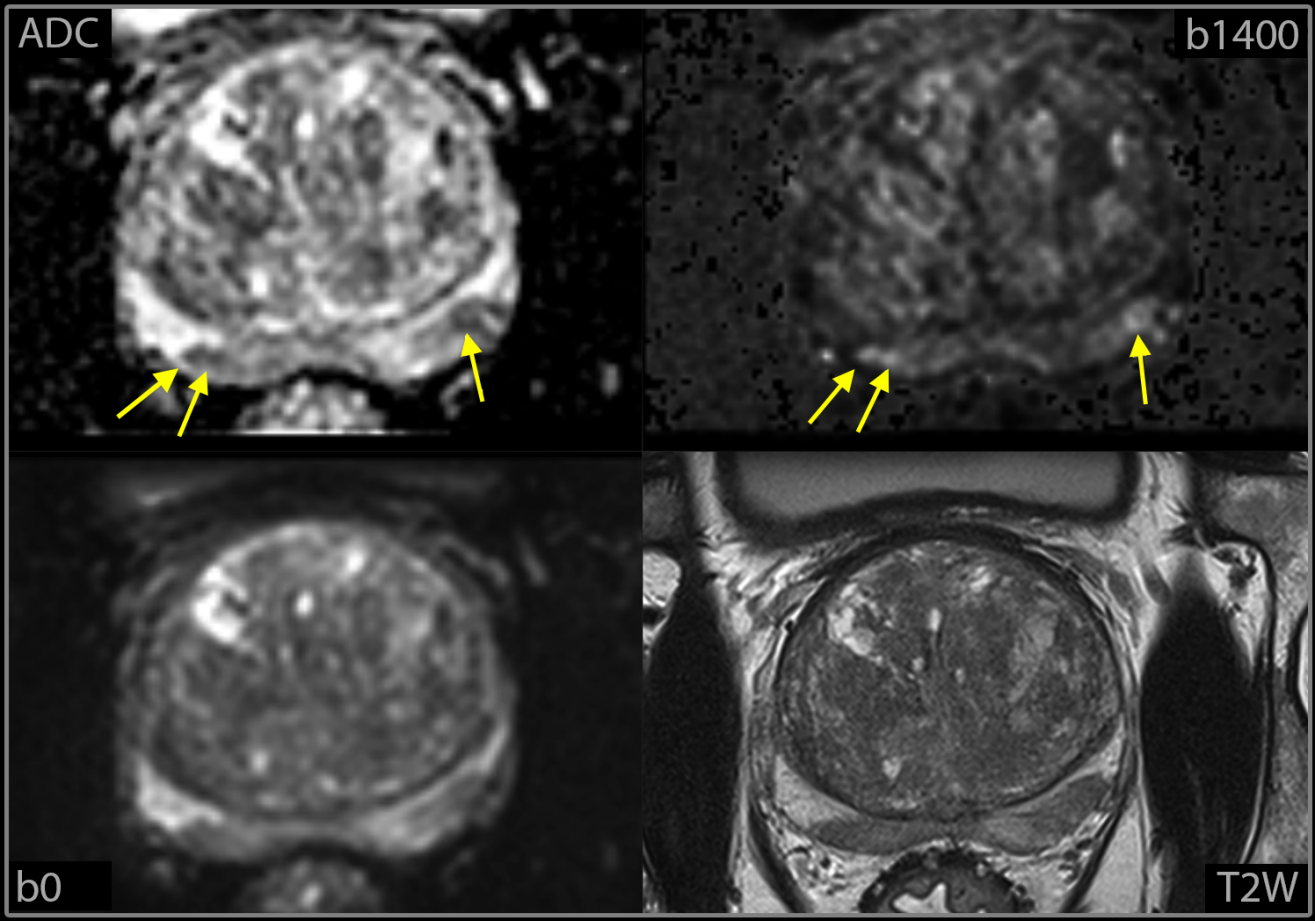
Example of small foci (yellow arrows) classified as equivocal at high-resolution DWI-only, with T2W sequence from mpMRI for comparison.
